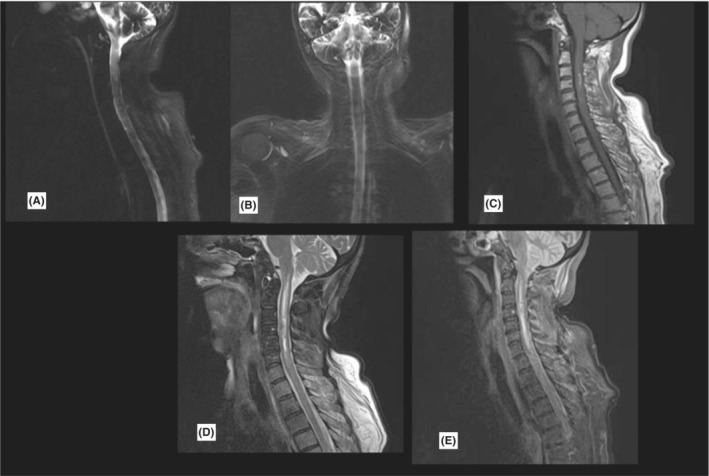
Cervicothoracic MRI demonstrates a hemorrhagic core corresponding to C3–C5 vertebral bodies with extensive cord edema originating from brain stem‐to‐upper thoracic cord myelography images showed no CSF block. A and B = myelograms, C = T1 sagittal view, sequence D = T2, sequence E = STIR image.
